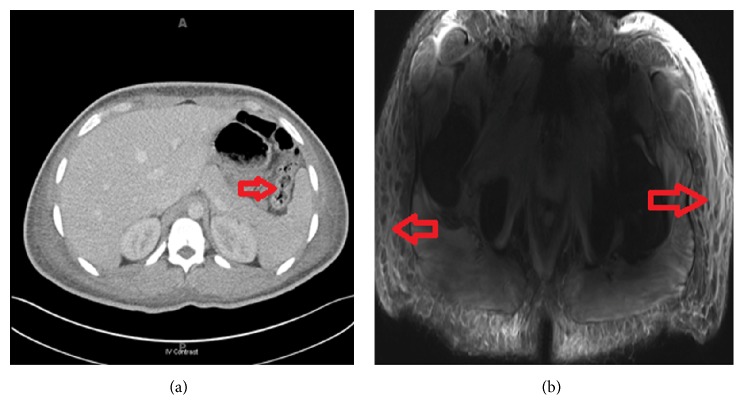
(a) CT of the abdomen showing thickening of the bowel wall (red arrow). (b) MRI showing edema of the soft tissue (red arrows).
